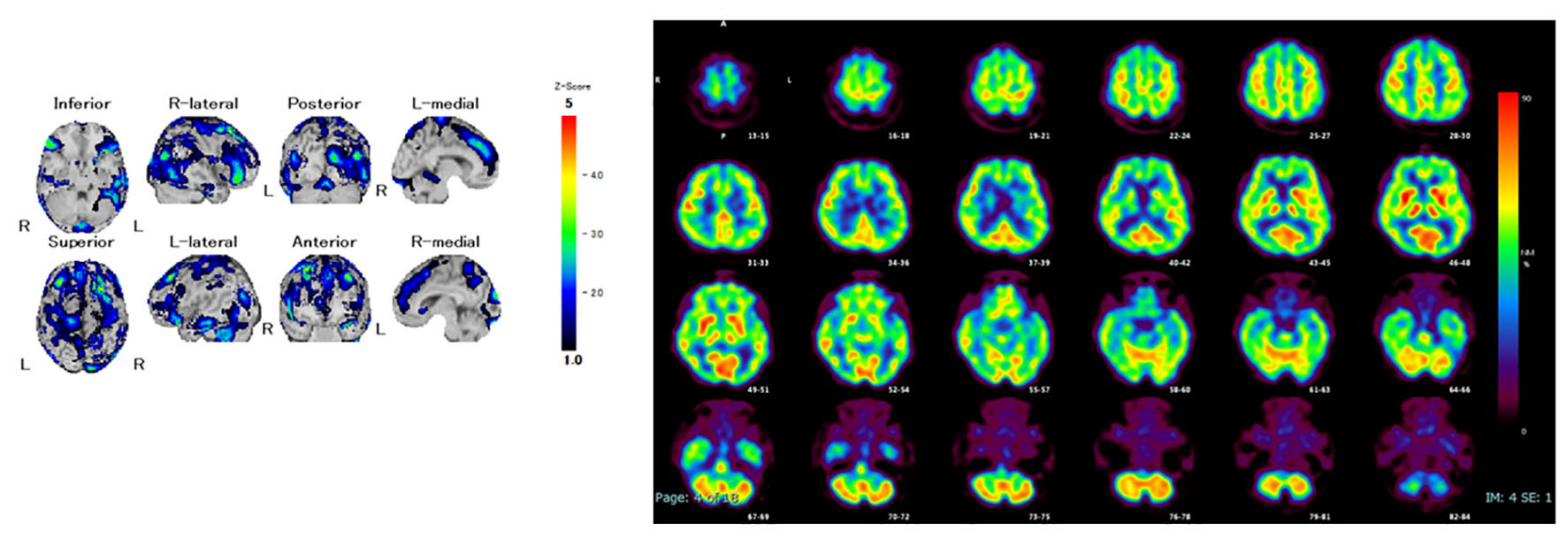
A single photon emission computed tomography.
